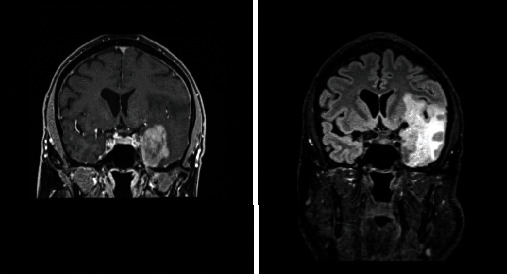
Four-centimeter extra-axial mass in the left temporal region. Radiographic differential diagnosis: meningioma.
